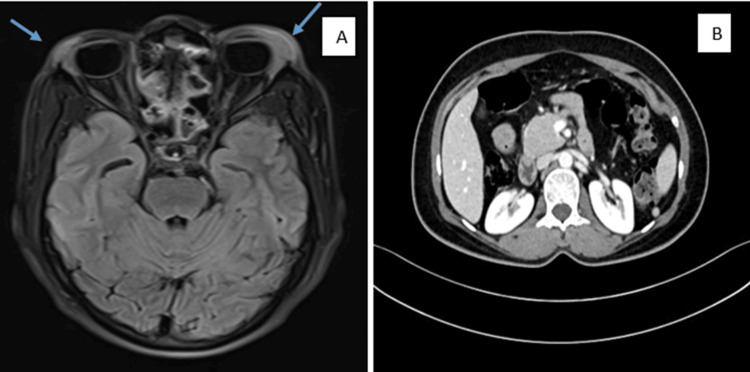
Radiological investigation (A) T2-FLAIR image of the brain, including the orbit, showing hyperintensity in both lacrimal glands (left more than right). (B) CECT of the whole abdomen showing no significant lymphadenopathy FLAIR, fluid-attenuated inversion recovery; CECT, contrast-enhanced CT
